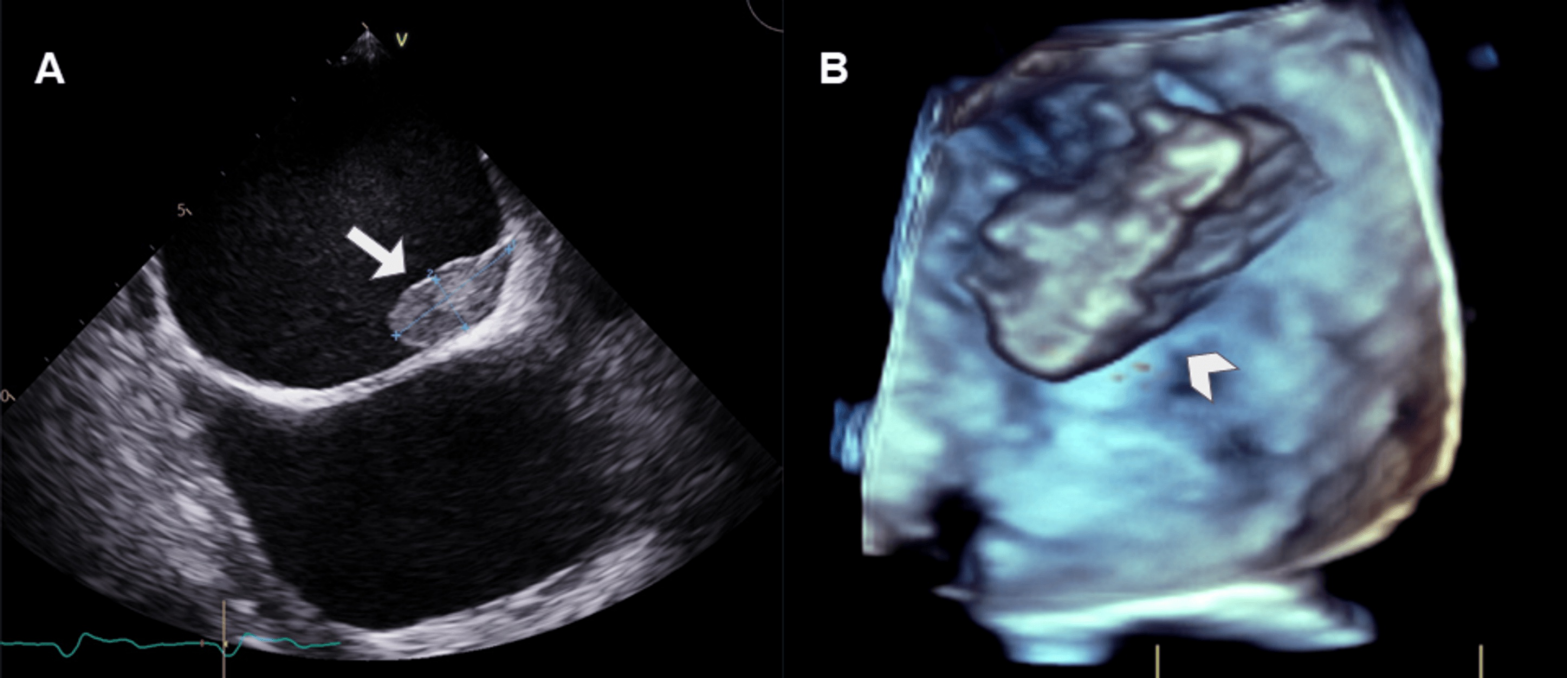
TEE in B mode (A) and 3D images in the left atrial (B). A woman presented with atrial fibrillation. TEE was performed and showed an ovoid lesion of intermediate echogenicity attached to the atrial roof (arrow in A). 3D echography images verify these findings (arrowhead in B) which are consistent with intracavitary thrombi. TEE: transesophageal echocardiography.
